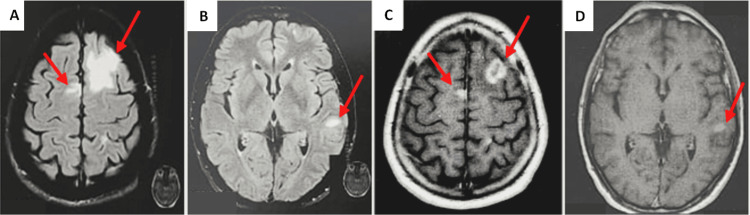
Axial fluid-attenuated inversion recovery-weighted (FLAIR) and post-contrast T1-weighted MRI of the brain demonstrating brain metastases. (A) Left anterior (right red arrow in image A) and right parasagittal (left red arrow in image A) frontal metastases, annular in appearance after contrast-enhancement (red arrows in image C) surrounded by a very large amount of finger glove edema clearly visible on FLAIR imaging (A) associated to an enhancing focus involving the left parietal lobe (red arrow in image B and D), consistent with intracranial metastatic disease.
